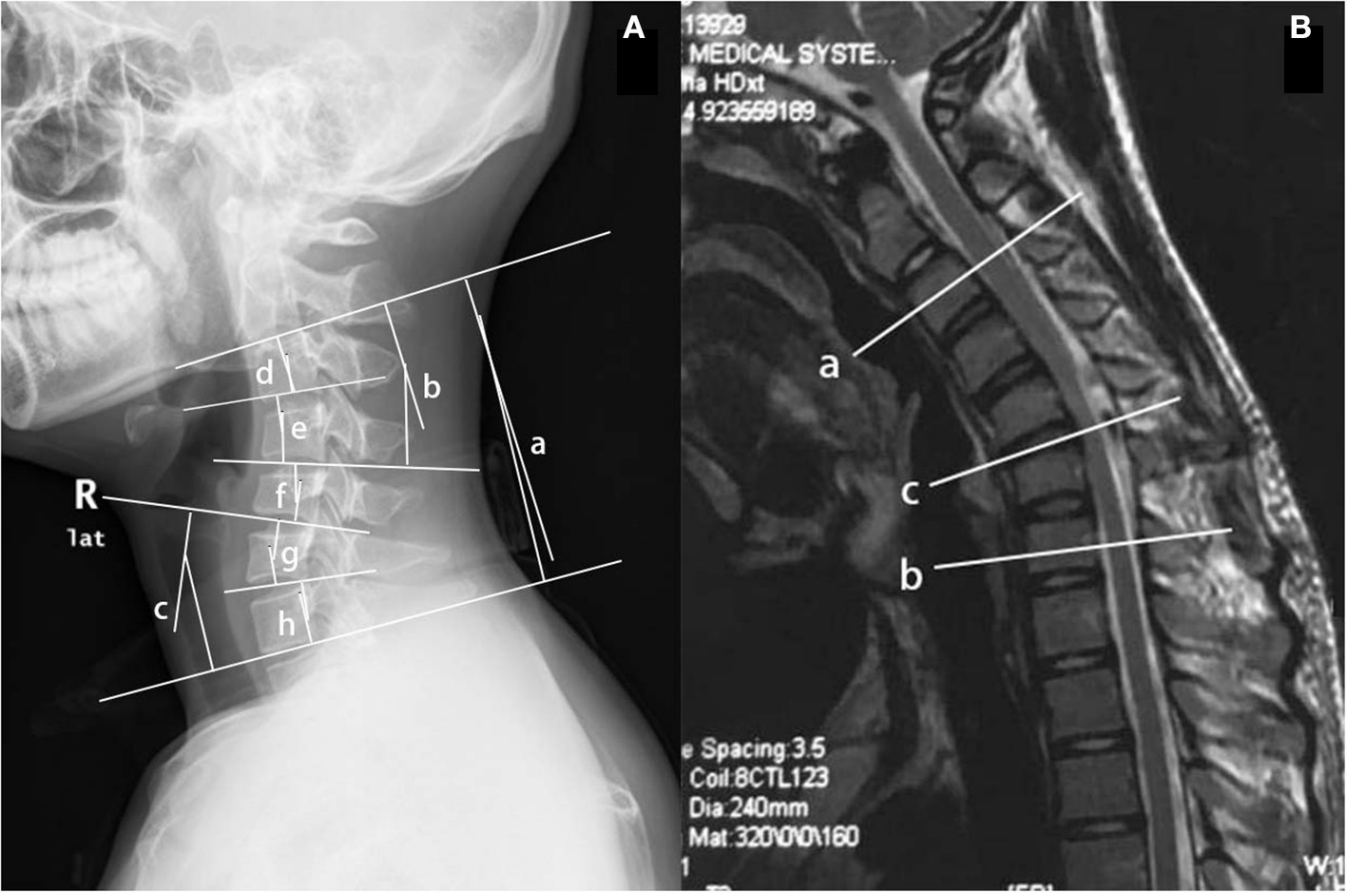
(A) The Cx-y Cobb angle was the angle between the two vertical lines of the two tangent lines under the inferior endplates of Cx and Cy on the conventional sagittal cervical radiograph. (a) C2-7 Cobb angle; (b) C2-4 Cobb angle; (c) C5-7 Cobb angle; (d) C2-3 Cobb angle; (e) C3-4 Cobb angle; (f) C4-5 Cobb angle; (g) C5-6 Cobb angle; and (h) C6-7 Cobb angle. (B) The affected segments and the most serious one on the sagittal cervical-flexion MRI. The upper end of the affected segments (a), the lower end (b), and the most serious segments (c) were recorded in the form of level of vertebral or intervertebral bodies corresponding to spinal cord segments.
